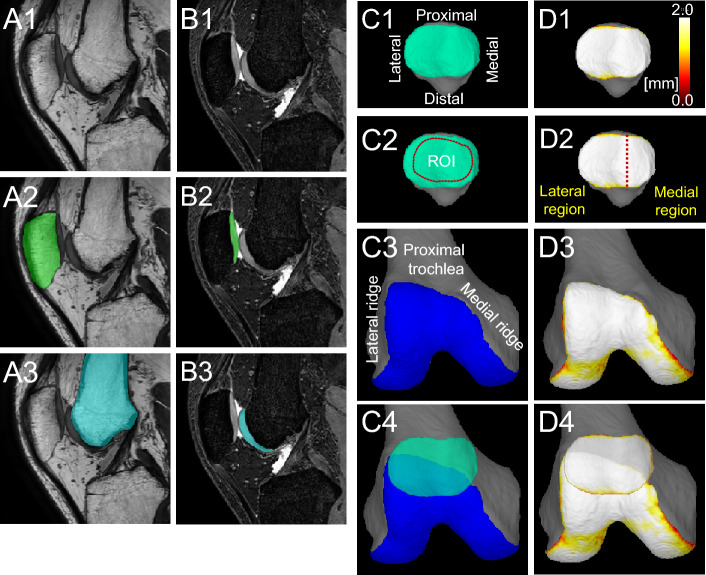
3D MRI analysis of the patellofemoral joint of a representative control knee on the right side. (A) Proton density–weighted (PDW) images of the sagittal plane. From the plain images (1), the bone regions were automatically segmented and colored green for the patella (2) and light blue for the femur (3). (B) Fat-suppressed spoiled gradient echo sequence (SPGR) images of the sagittal plane. From the plain images (1), the cartilage regions were automatically segmented and colored green for the patella (2) and light blue for the femur (3). (C) 3D images of the PF cartilage. The patellar cartilage surface of the right knee is shown inverted from left to right (1). Inner margins of 2 mm of the original or predicted original patellar cartilage are set as the ROI (2). The long axis of the femur is tilted 45°, and the anterior surface of the femoral trochlear cartilage is shown in blue (3). The patellar cartilage is superimposed on the femoral trochlear cartilage (4). (D) Cartilage thickness mapping of the PF joint. Patellar cartilage thickness is shown in color (1). The medial 2/5 of the patellar cartilage is defined as the medial region, and the lateral 3/5 as the lateral region (2). The femoral trochlear cartilage thickness is shown in color (3). The patellar cartilage thickness map is superimposed on the femoral trochlear cartilage thickness map (4).
